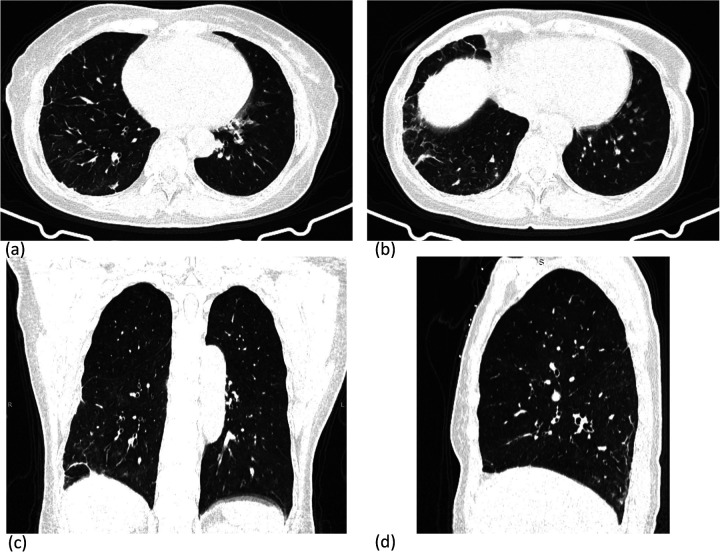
Chest CT revealed linear opacities in the right middle and lower lobes with interlobular septal thickening, suggestive of pulmonary interstitial changes (a,b) axial; (c,d) coronal reconstruction.
